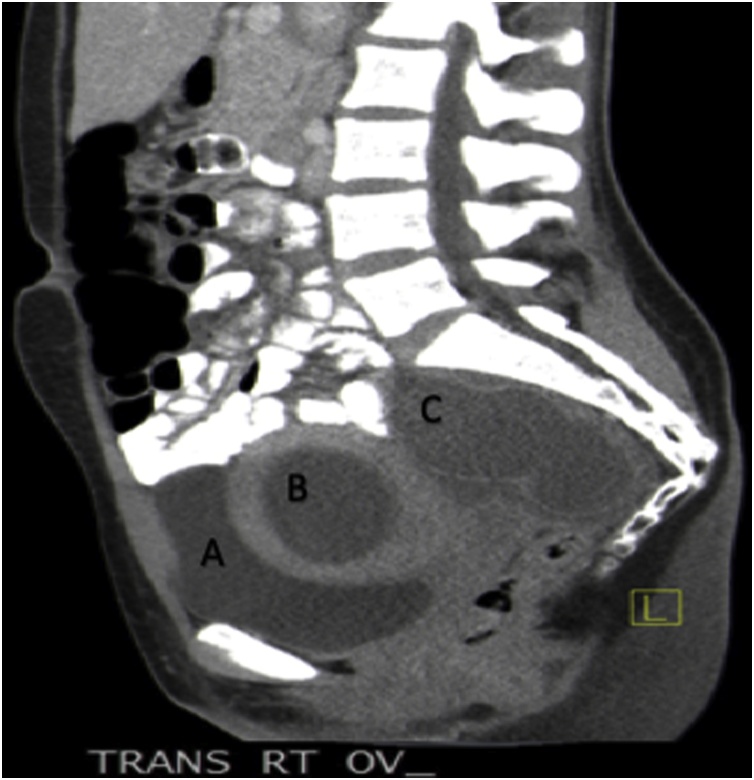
The sagittal plane of CT scan with oral contrast: A. Bladder, B. Hematometra in the non-communicating rudimentary horn with active cavity, misread as right ovarian complex mass, C. Right dilated fallopian tube.
